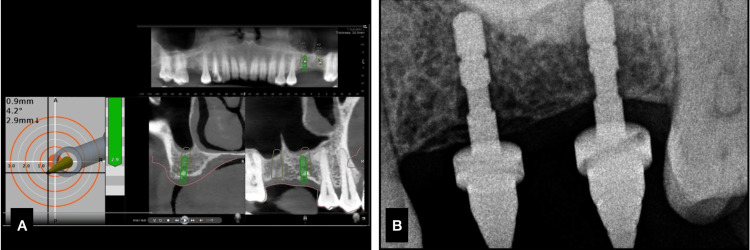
Case 1. A) Drilling under Dynamic navigation, B. position indicating device (PID) radiograph.
